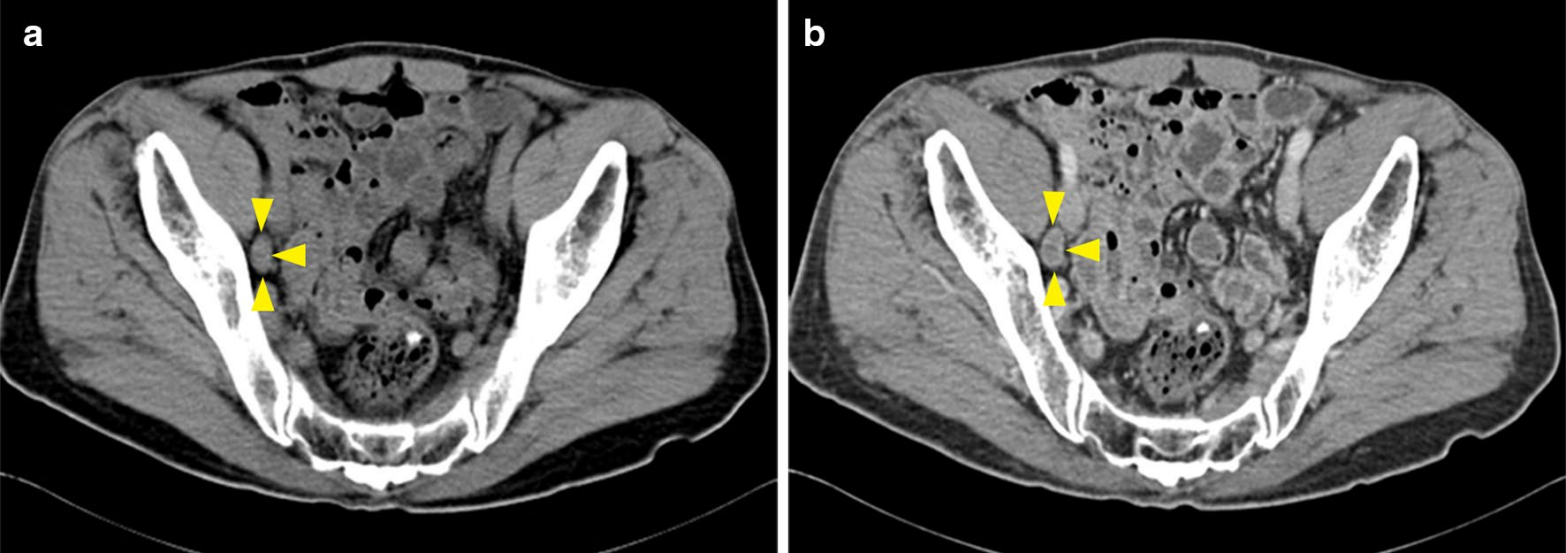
Pelvic computed tomography (CT) images of a 76-year-old man with bladder cancer (stage pT3a). a CT scan before contrast shows an isodensity oval lymph node with a short-axis diameter of 9.0 mm in the right obturator region (yellow arrows). b In the vein phase after contrast, the necrosis in the center of the lymph node shows annular enhancement (yellow arrows). This lymph node with necrosis was proved to be metastatic in pathologic diagnosis
